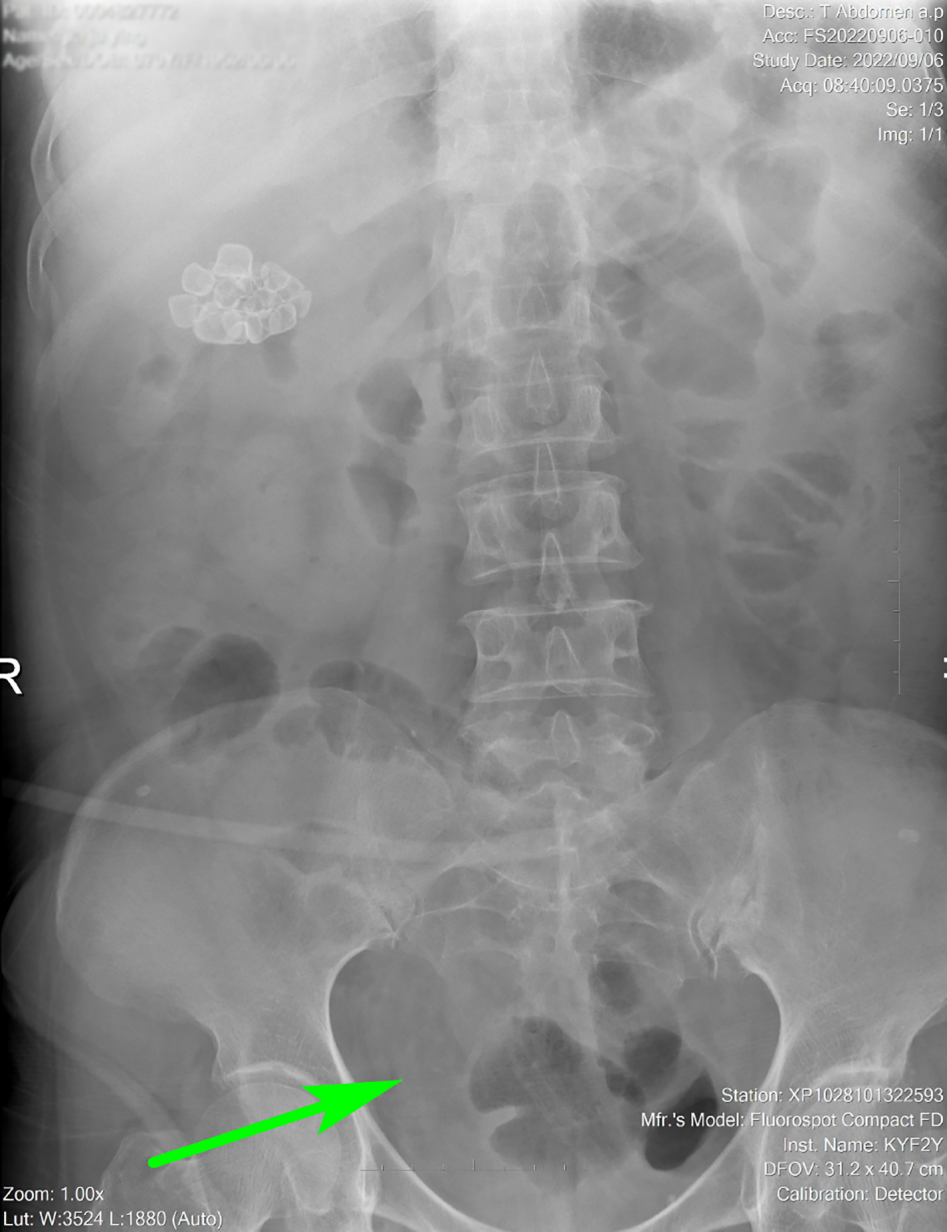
Postoperative abdominal x-ray showing no stones (green arrow).
